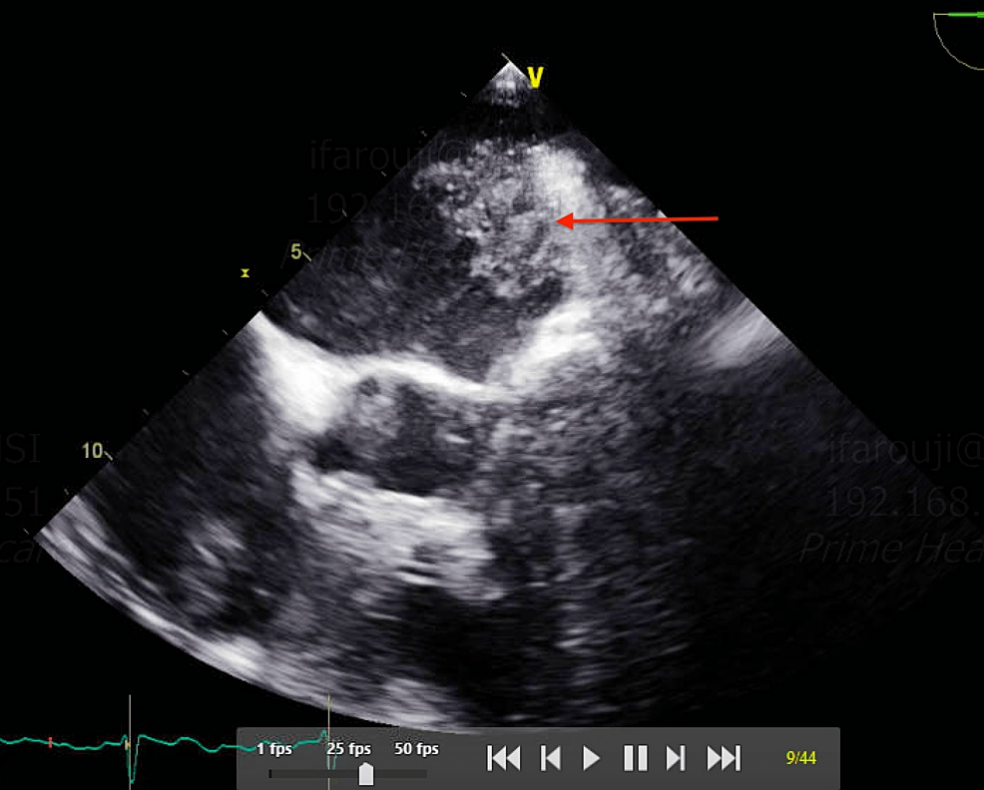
TEE showing a thrombus attached to the interatrial septum from the right side (red arrow) TEE: transesophageal echocardiogram
